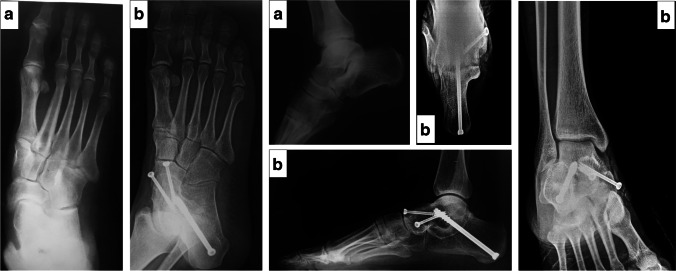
Pre-operative (a) and 12 months post-operative (b) radiographs of a double arthrodesis. This 26-year-old male patient with right-sided PTTI stage III had a significant improvement in his AOFAS hindfoot score from 60 pre-operatively to 90 post-operatively. Union was achieved at 3.5 months. No complications were reported, and the surgical time was 70 min
